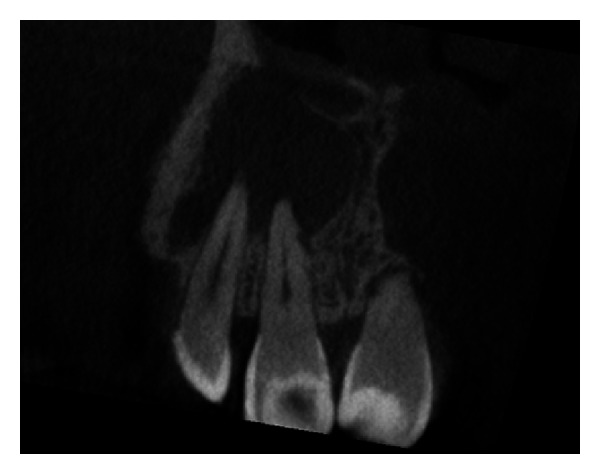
Pre-operative CBCT image in coronal plane showing presence of a large periapical lesion.
